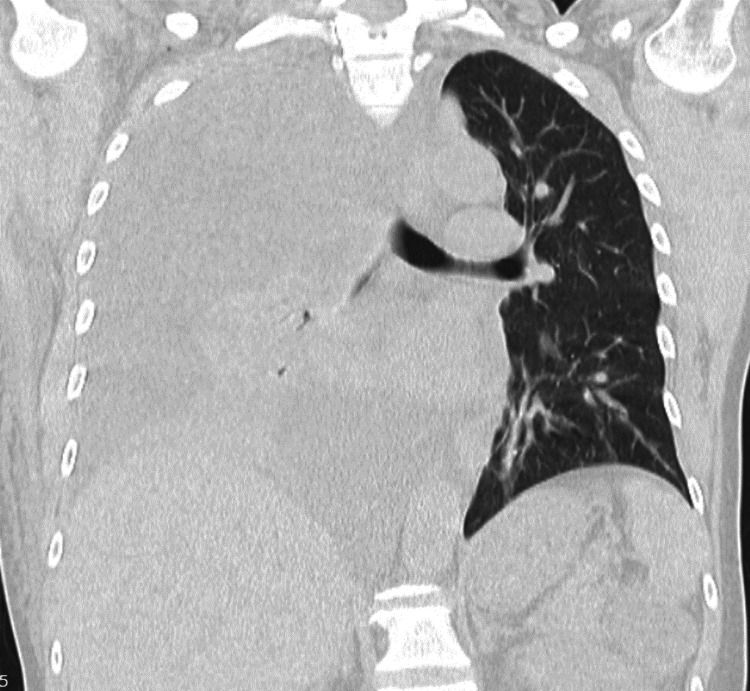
Chest computed tomography with a large right-sided pleural effusion causing contralateral mediastinal shift and nearly complete passive atelectasis of the right lower lobe
